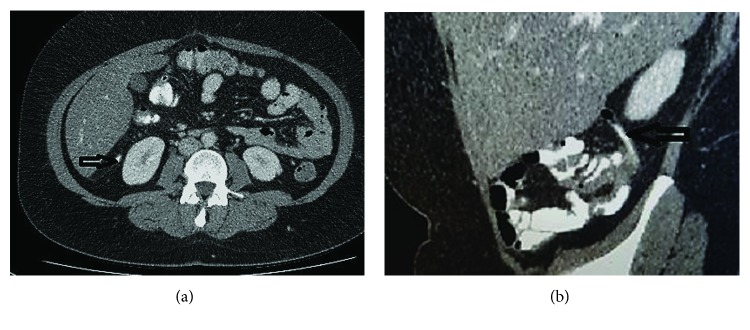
(a) Axial CT abdomen image and (b) sagittal image. The appendix looked entirely normal (horizontal arrows in (a) and (b) images).
